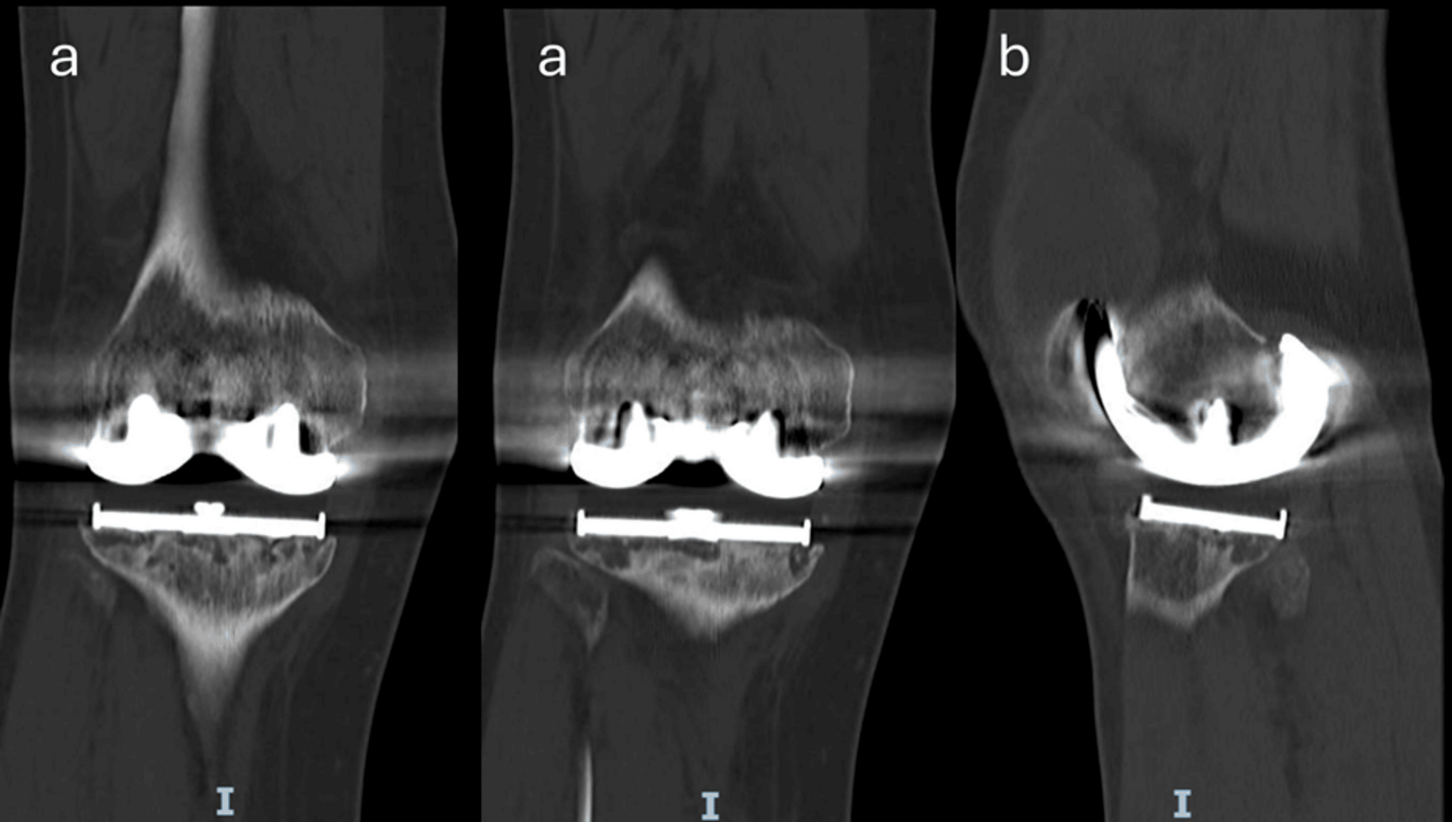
Computed tomography (CT) views of the right knee demonstrating lucency around the tibial tray. (a) Coronal views showing lucency at the tibial tray; (b) Sagittal views demonstrating lucency at the posterior aspect of the tibia tray
